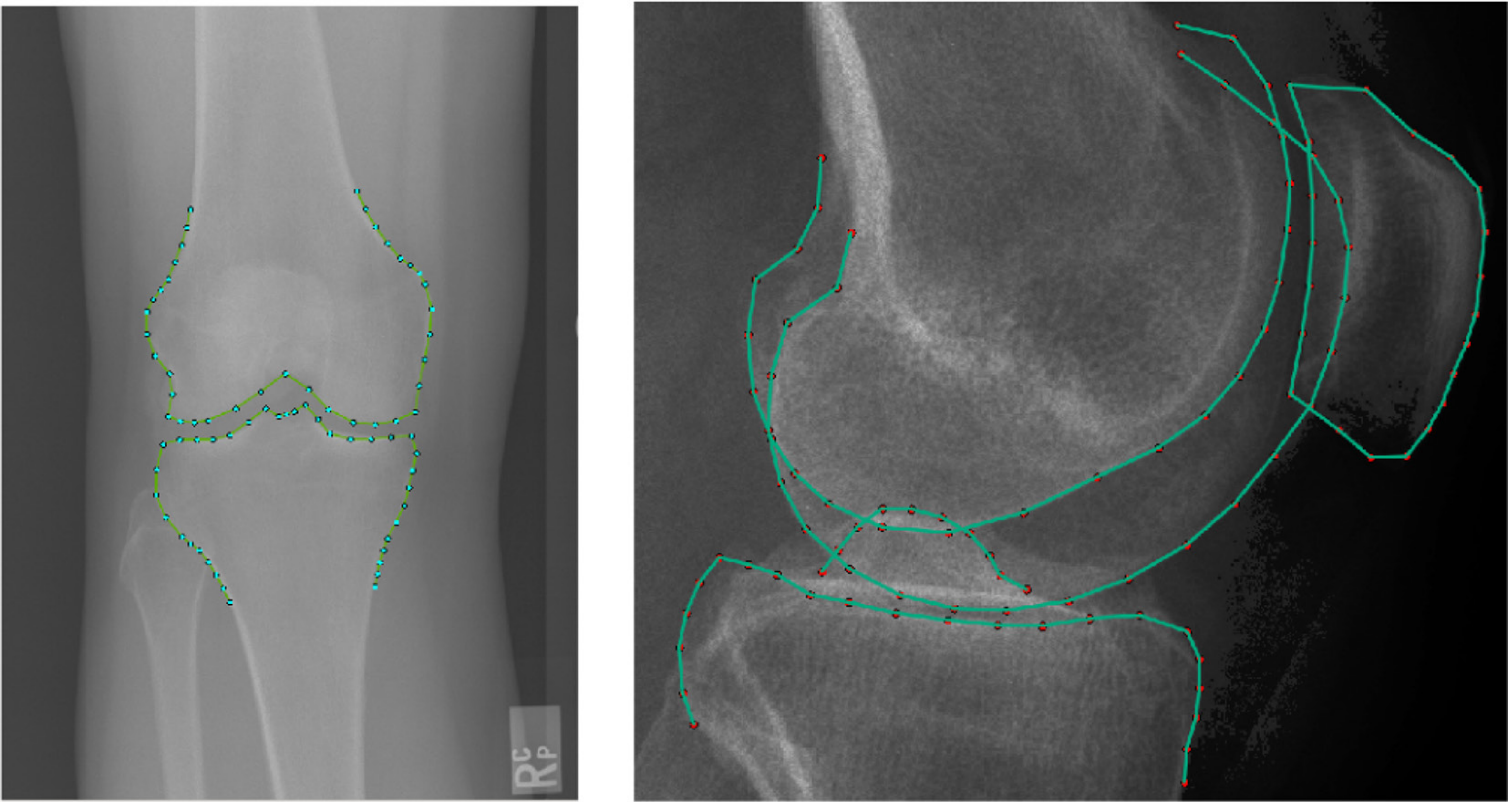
Our model for lateral radiographs (right) was made of four subshapes: the patella (21 points), the lateral femoral condyle (24 points), the medial femoral condyle (25 points) and the tibia (32 points). We considered the femur as the union of the two femoral condyles (49 points). The PA model (left) was made of two shapes: the femur and the tibia (37 points each, a total of 74 points).
